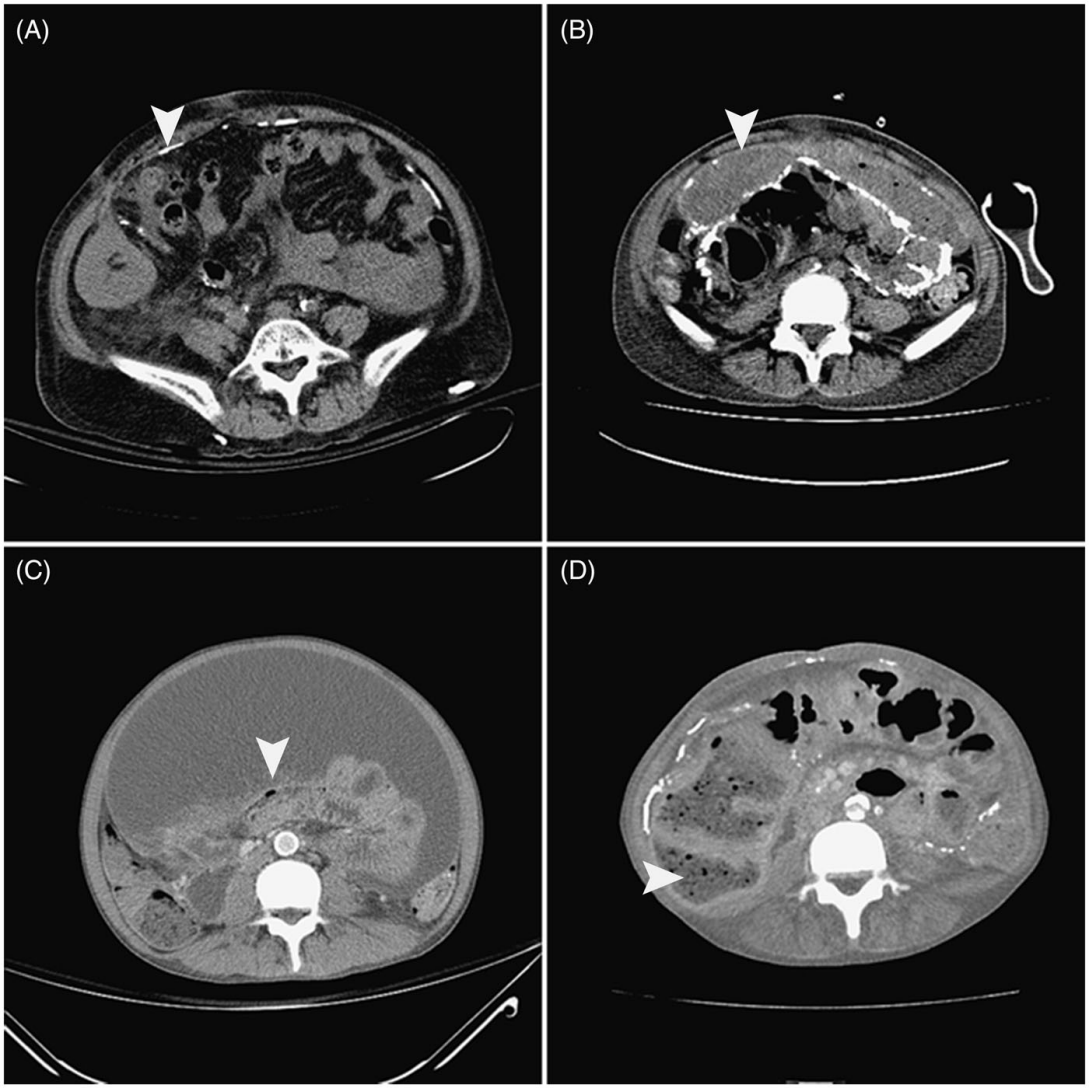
Cross-sectional abdominal computed tomography images of patients diagnosed with EPS. Note the peritoneal calcification (A, arrow), peritoneal thickening surrounding the ascites (B, arrow), bowel tethering (C, arrow), and bowel dilation (D, arrow) in the patients who discontinued peritoneal dialysis.
